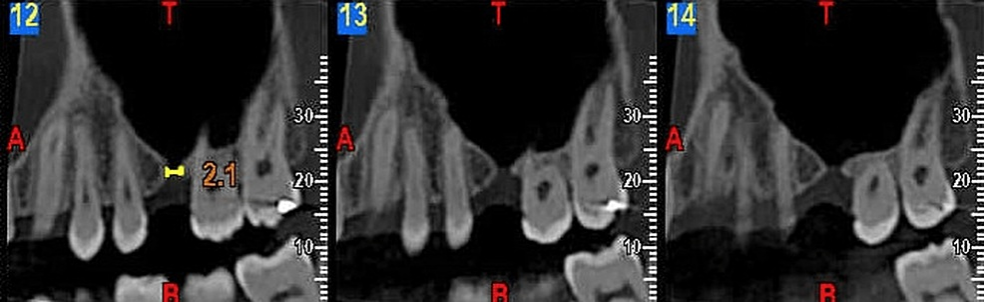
CBCT imaging of the lesion
